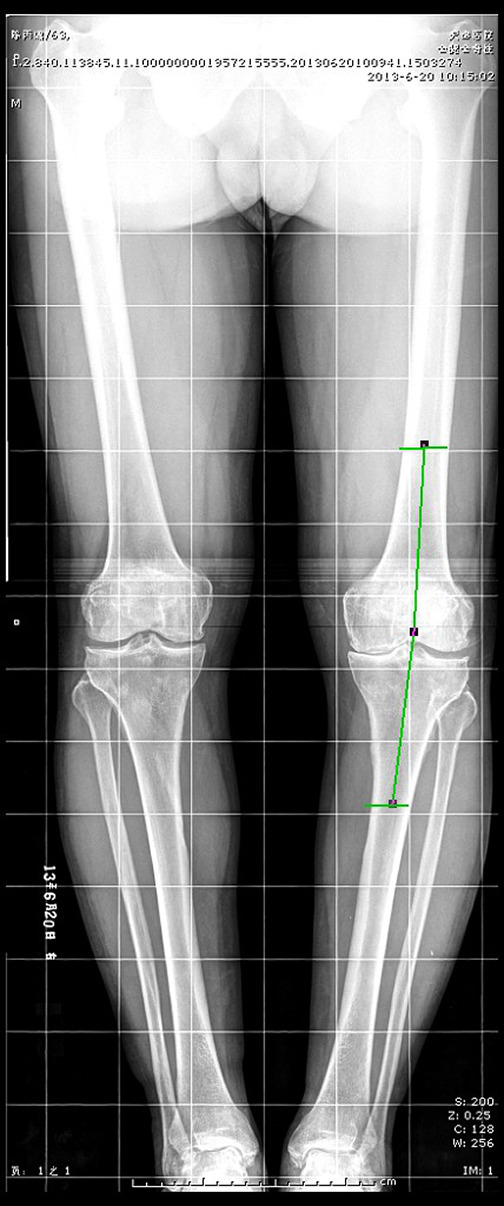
Full‐length Radiography of Both Lower Limbs at Weight‐bearing Position.
